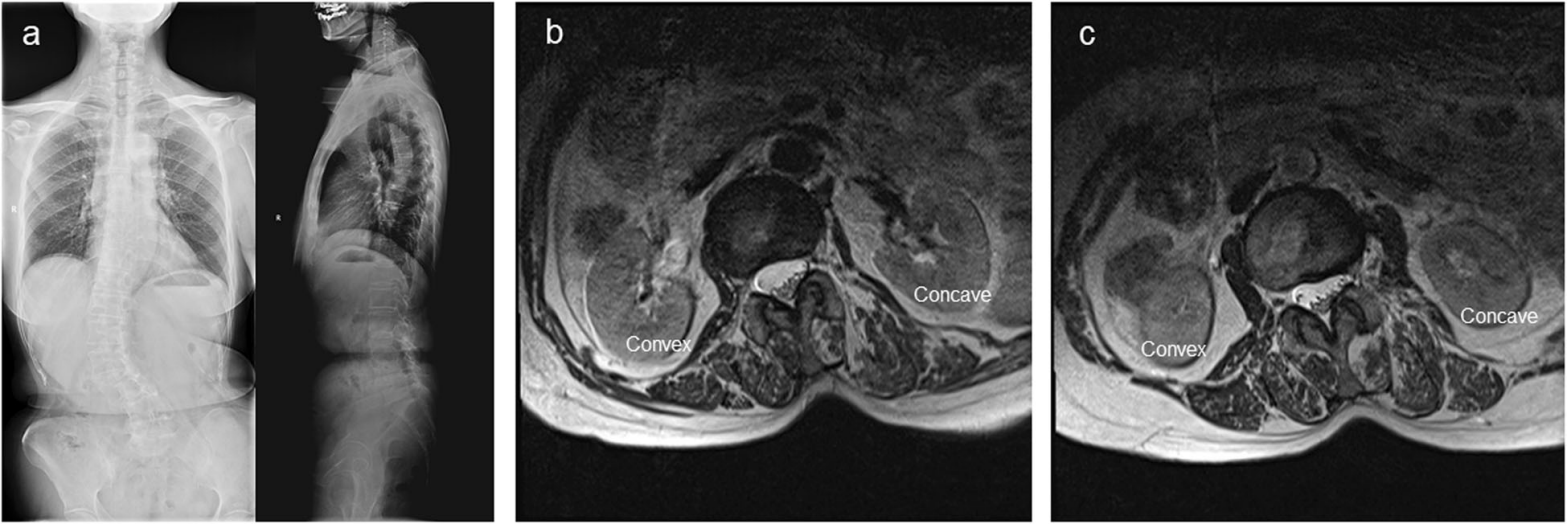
A 58-year-old female patient with adult degenerative scoliosis (ADS). a Standing anteroposterior (AP) and lateral radiographs. Coronal Cobb angle (CA), 35.6°; coronal vertical axis (CVA), − 1.6 cm; sagittal vertical axis (SVA), + 4.8 cm; thoracic kyphosis (TK), 9.2°; lumbar lordosis (LL), 28.1°; pelvic incidence (PI), 62.6°; pelvic tilt (PT), 35.2°; sacral slope (SS), 27.4°. b T2-weighted axial image at intervertebral levels above the apical vertebra (T12/L1): grade 1 LMA on the convex side and grade 4 LMA on the concave side. c T2-weighted axial images at the intervertebral level below the apical vertebra (L1/2): grade 2 LMA on the convex side and grade 3 LMA on the concave side
